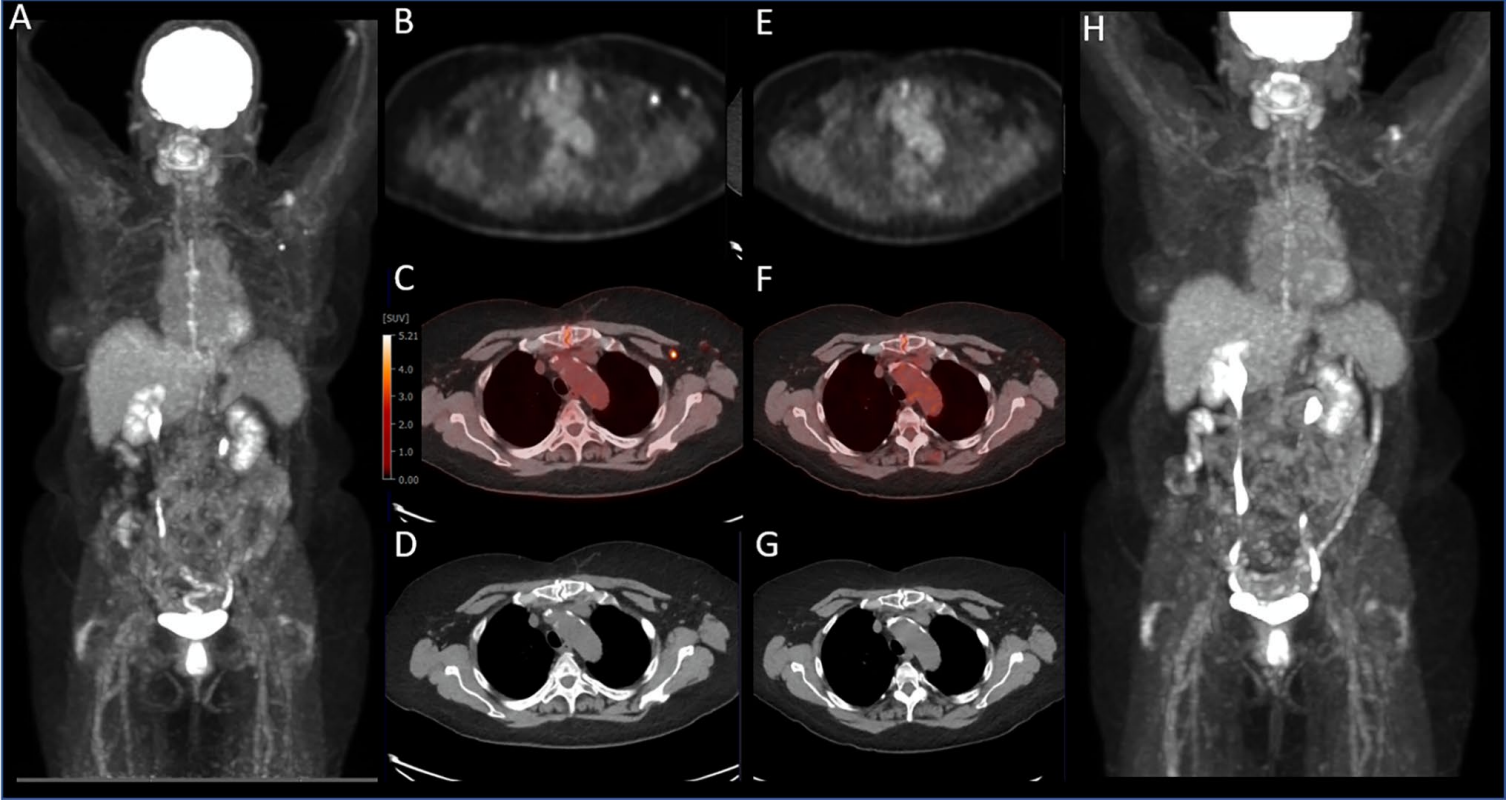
Selected images from serial FDG PET/CT examination for a patient who received 1 dose of Pfizer vaccination 11 days before initial imaging, as well as a second FDG PET/CT approximately 3 months later for clinical oncologic follow-up, with interval administration of the second dose of COVID-19 vaccination. FDG PET MIP (A) trans-axial FDG PET (B) fused FDG PET/CT (C) and trans-axial non-contrast CT images show a non-enlarged but hypermetabolic left axillary lymph node; ΔSUVmax = 5.9. On the subsequent evaluation, FDG PET MIP (H) trans-axial FDG PET (E) fused FDG PET/CT (F) and trans-axial non-contrast CT images FDG PET (G) images at matching levels show resolution of FDG uptake in the same-sized lymph node, now seen only on the CT image
